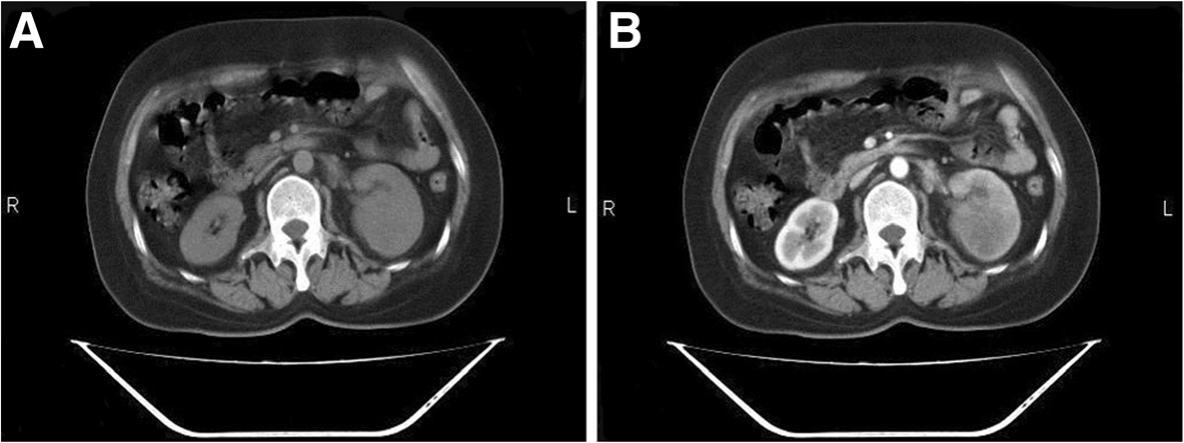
NSCLC renal metastasis. Computed tomography scan (A) and enhanced scan (B).
